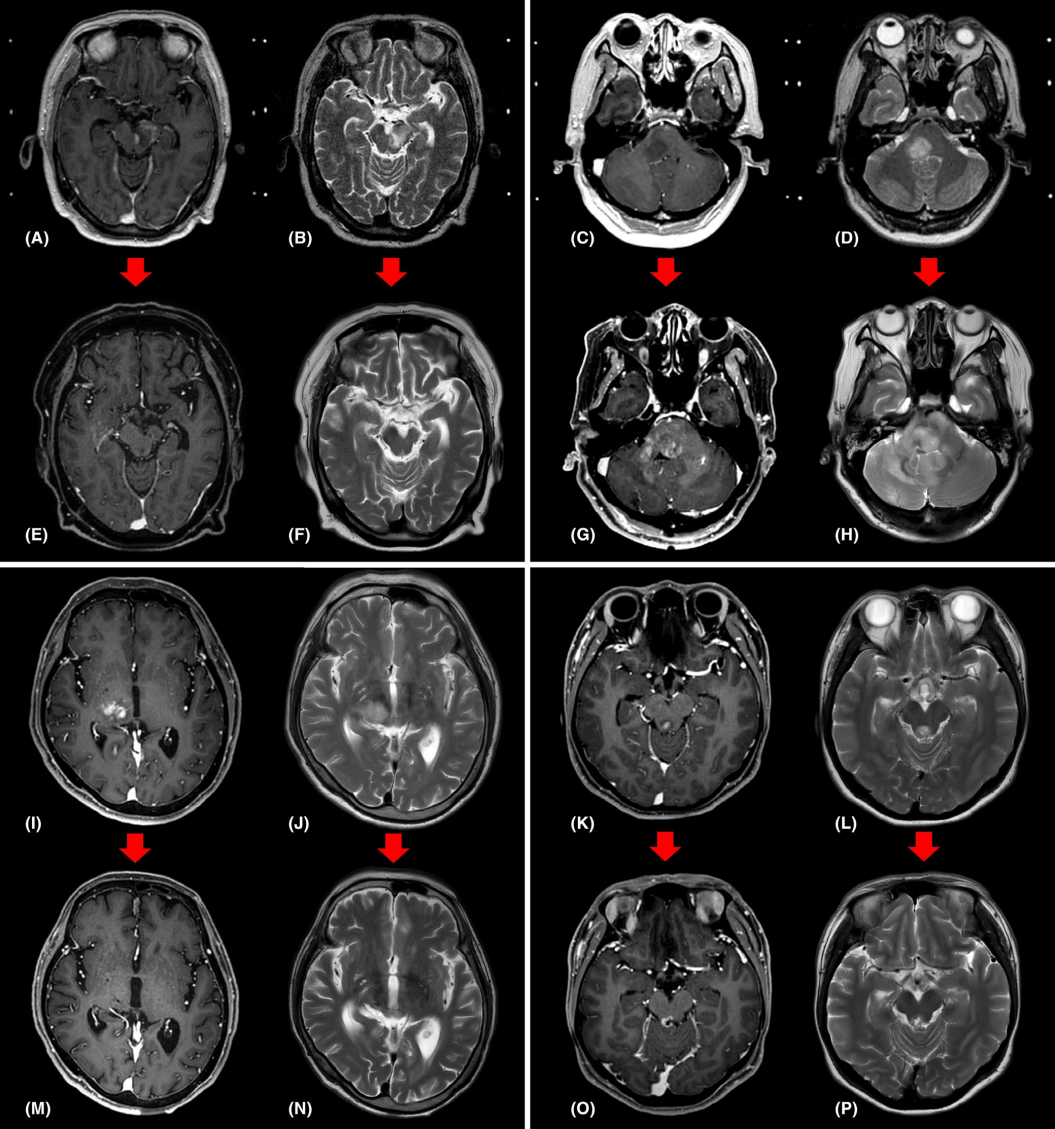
MRI findings for two cases with different radiological and pathological diagnoses and two cases with same radiological and pathological diagnoses. (A, B) T1‐weighted image with gadolinium enhancement and T2‐weighted image of a 62‐year‐old woman complaining of hemiparesis. A T2 hyperintense lesion with subtle focal enhancement is located in the left midbrain. Radiologically, low grade glioma with high‐grade component was suspected, but it was diagnosed as a demyelinating disease through stereotactic biopsy. (E, F) The patient was treated with steroids, and the MRI taken 3 months later showed improvement of the lesion. (C, D) T1‐weighted image with gadolinium enhancement and T2‐weighted image of a 50‐year‐old woman complaining of dizziness. An infiltrative T2 hyperintense lesion without enhancement is located in the right pons and medulla. Radiologically, low grade glioma was suspected, but diffuse midline glioma was diagnosed through stereotactic biopsy. (G, H) Despite concurrent chemoradiation therapy, the MRI taken 2 years later showed disease progression. (I, J) T1‐weighted image with gadolinium enhancement and T2‐weighted image of a 72‐year‐old woman complaining of hemiparesis. A T2 high signal intensity lesion with enhancement is located in the right thalamus, midbrain, and pons. Although lymphoma was suspected by radiology, it was necessary to exclude demyelinating lesion or high‐grade glioma. Diffuse large B cell lymphoma was confirmed through stereotactic biopsy, and chemotherapy (Methotrexate, Vincristine, and Dexamethasone) was initiated. (M, N) The enhancing lesion disappeared on follow‐up MRI after 6 months. (K, L) T1‐weighted image with gadolinium enhancement and T2‐weighted image of a 43‐year‐old woman complaining of diplopia. A contrast‐enhancing lesion is observed in the midbrain. High‐grade glioma was suspected by radiology, and pathological diagnosis was attempted through stereotactic biopsy to determine the treatment. After confirmation of anaplastic astrocytoma, the patient underwent radiation therapy. (O, P) And there was no significant change in follow‐up MRI after 6 months. MRI, magnetic resonance imaging
